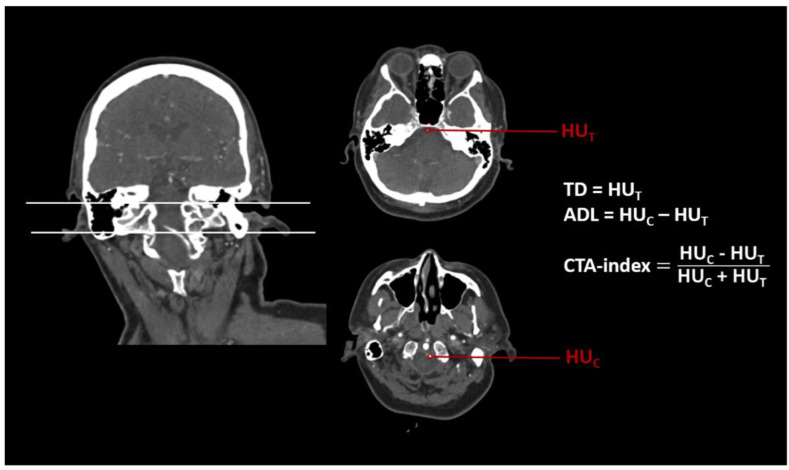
Example of an assessment of CT imaging markers pure thrombus density (TD), absolute density loss (ADL), and CTA-index (relative thrombus attenuation) for a patient (male, 60 years) with an acute basilar artery occlusion. ROIs for measurements of mean Hounsfield Units (HU) were placed at the occlusion site (T) and a reference point (C).
